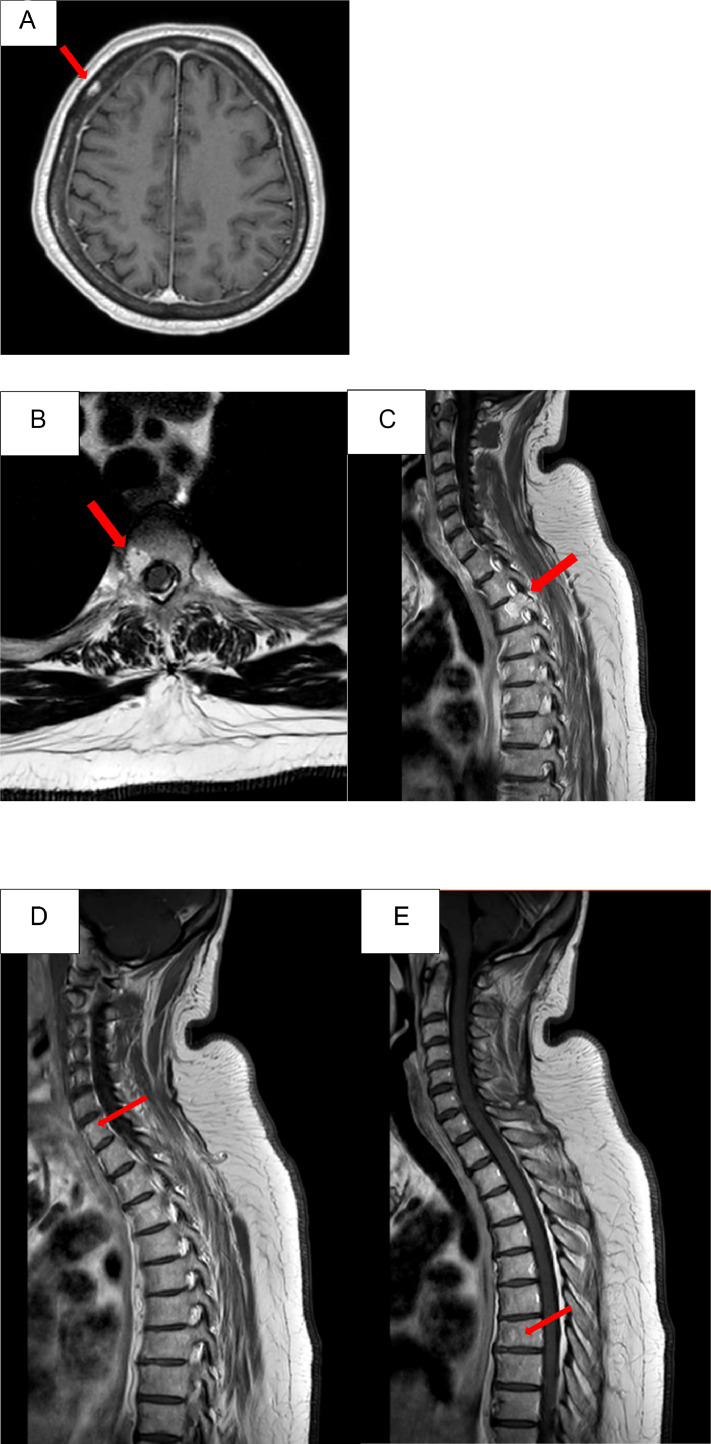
(A) Axial view: T1-weighted gadolinium enhanced MR shows small angioma in the right frontal theca. (B-C)*Sagittal view*: T1-weighted gadolinium enhanced MR and *axial view* T2 MR: show an angioma of the posterior upper aspect of D3 extending to the vertebral peduncle, the transverse process and part of the ipsilateral lamina. D-E)*Sagittal view*: T1-weighted gadolinium enhanced MR: they show a millimetric angioma at the level of C7 and D7, respectively.
